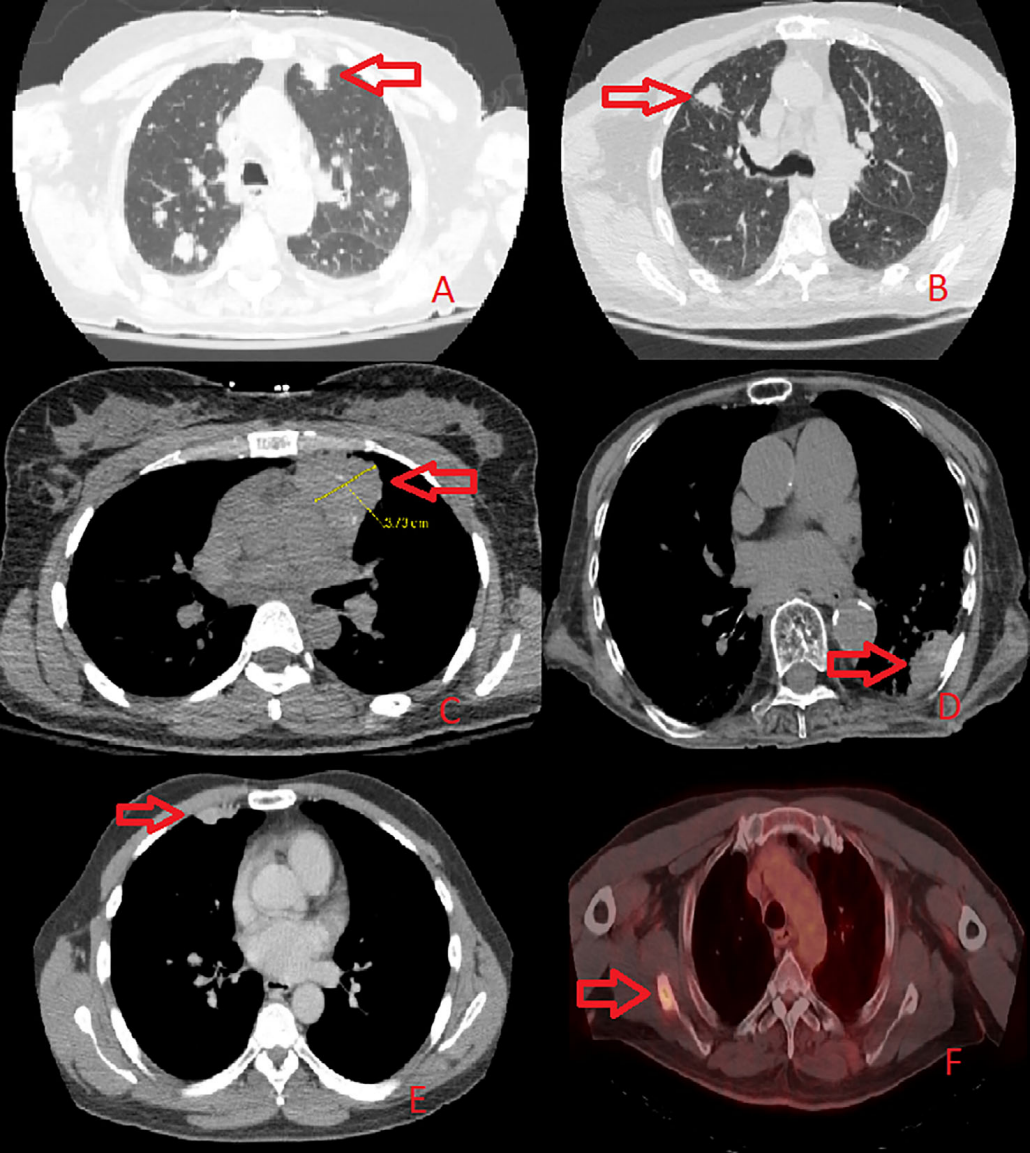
Sample computed tomography images of the six masses where fiducials were implanted (A: Patient 1; B: Patient 2; C: Patient 3; D: Patient 4; E: Patient 5; F: Patient 6)
